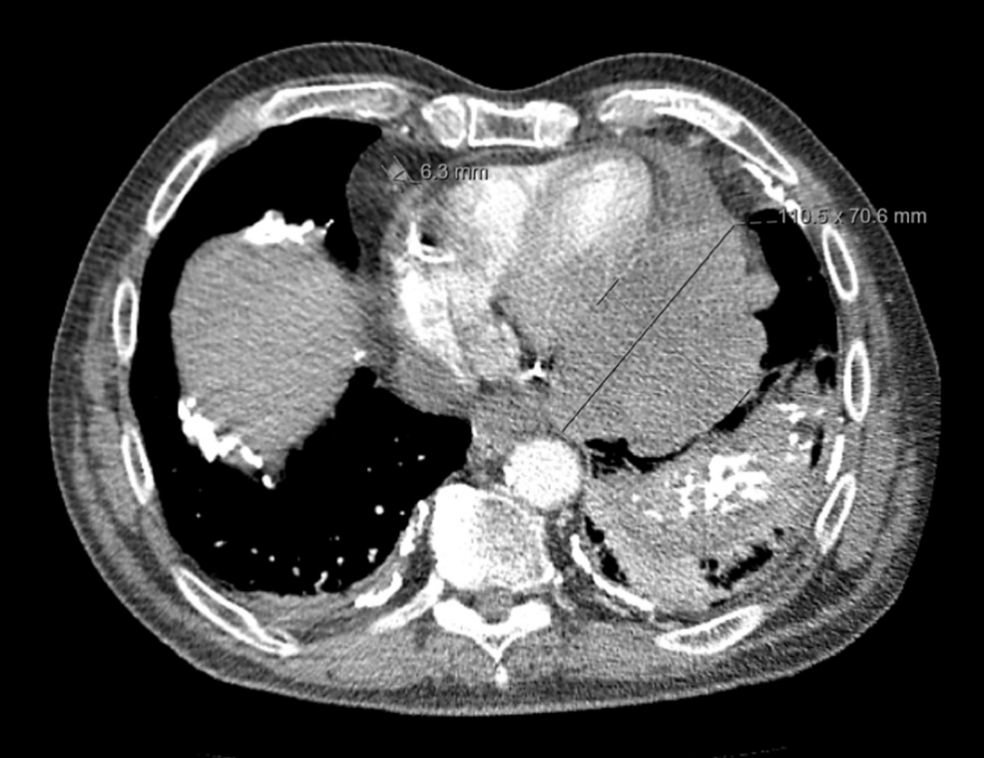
Cross-sectional CT showing pericardial mass measuring 110.5 x 70.6 mm Pleural plaques are present on the inferior aspect of the left lung.
